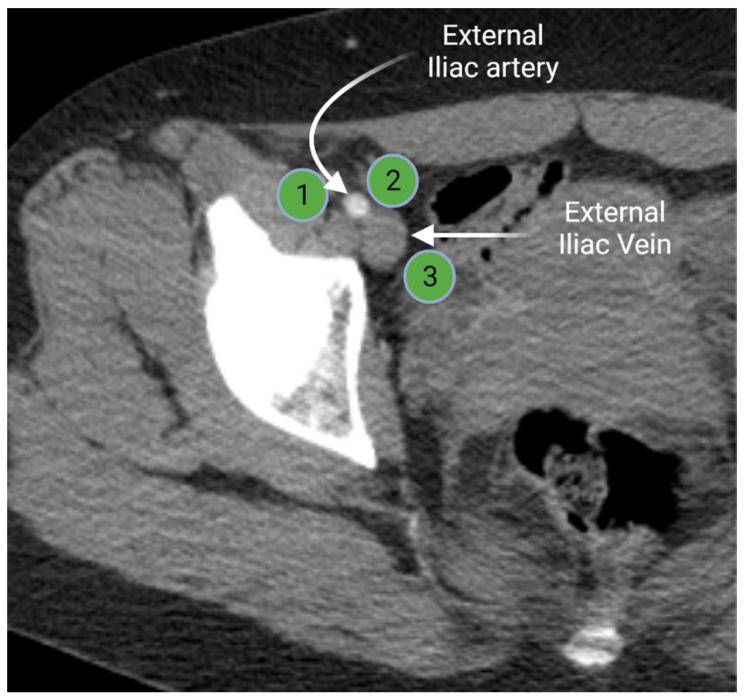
Axial contrast-enhanced computed tomography of the right hemipelvis demonstrates the right external iliac artery and veins with associated lateral (1), middle (2), and medial (3) external iliac lymph nodes. “Created by BioRander.com. accessed on 10 August 2021”.
